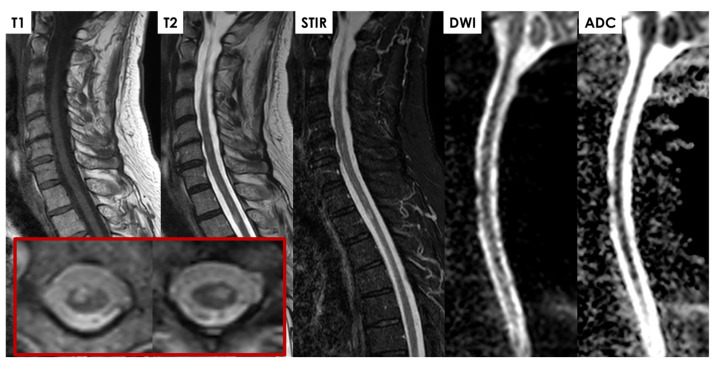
A 78-year-old patient with multiple vascular risk factors underwent vascular surgery for a thoraco-abdominal aortic aneurysm, with complications during awakening due to inability to extubate, which was performed three days later. After extubation and awakening, hyposthenia was noted in all four limbs, asymmetric (but predominantly affecting the lower limbs), with areflexia in the lower limbs and hypo-responsive proprioceptive reflexes in the upper limbs. The patient was catheterized preoperatively, with sphincter function not fully assessable. Sensory levels were unclear. An MRI study was performed 5 days post-surgery under the best possible conditions (hemodynamically stable patient but in fragile balance, on high-flow therapy, GFR < 30 mL/min, etc.). Multifocal intramedullary lesions in the high cervical–thoracic segment (at least 4) were hyperintense on T2 with different lateralization on axial scans (red lined figures) and involvement of the anterior horns (the left axial image corresponds to the highest lesion at C6-C7, and the right one to the lesion at the D3 level). DWI/ADC findings are consistent with timing (5 days post-surgery). Contrast administration was not performed due to renal failure, and the non-contrast study was already diagnostic given the clinical context.
